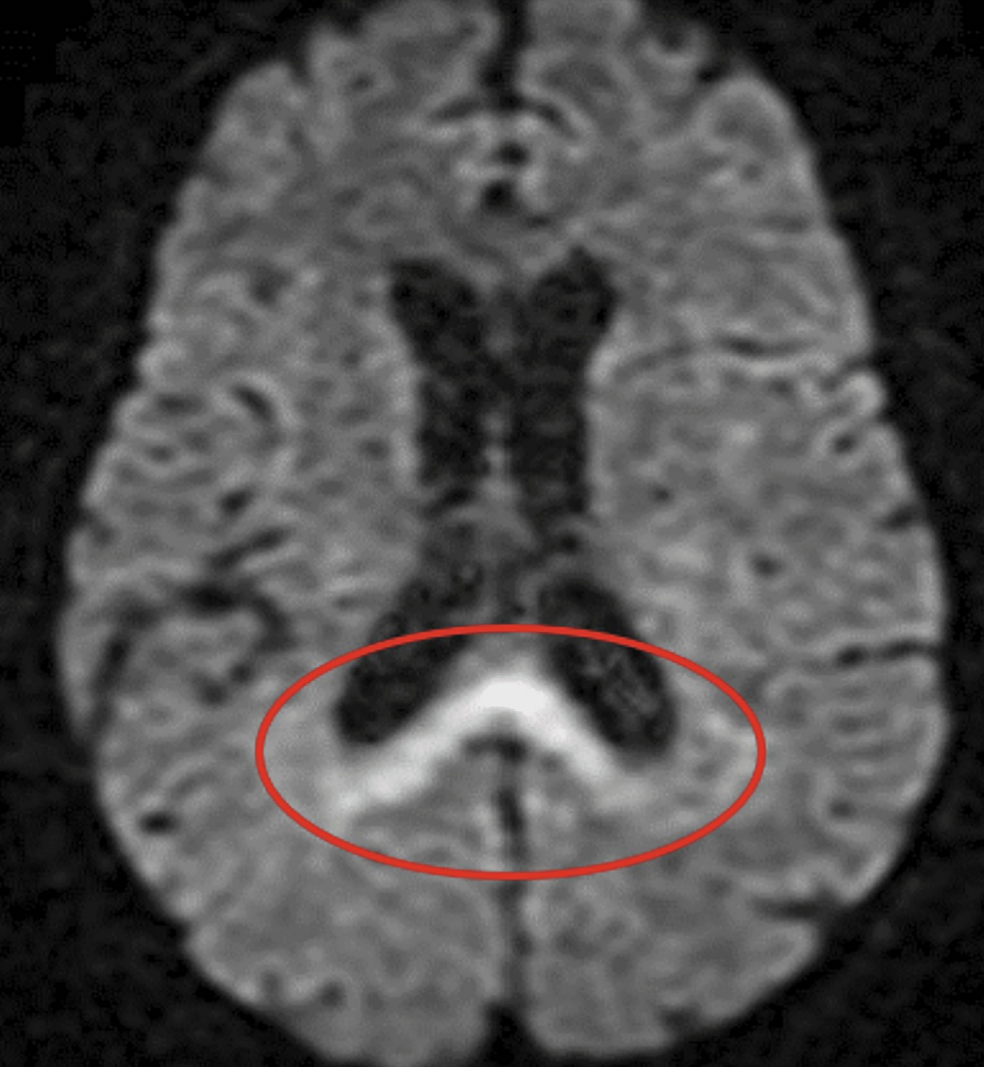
Brain MRI showing an abnormal DWI signal in the posterior corpus callosum in a patient with a positive GAD antibody (red circle). DWI: diffusion-weighted imaging; GAD: glutamic acid decarboxylase.
